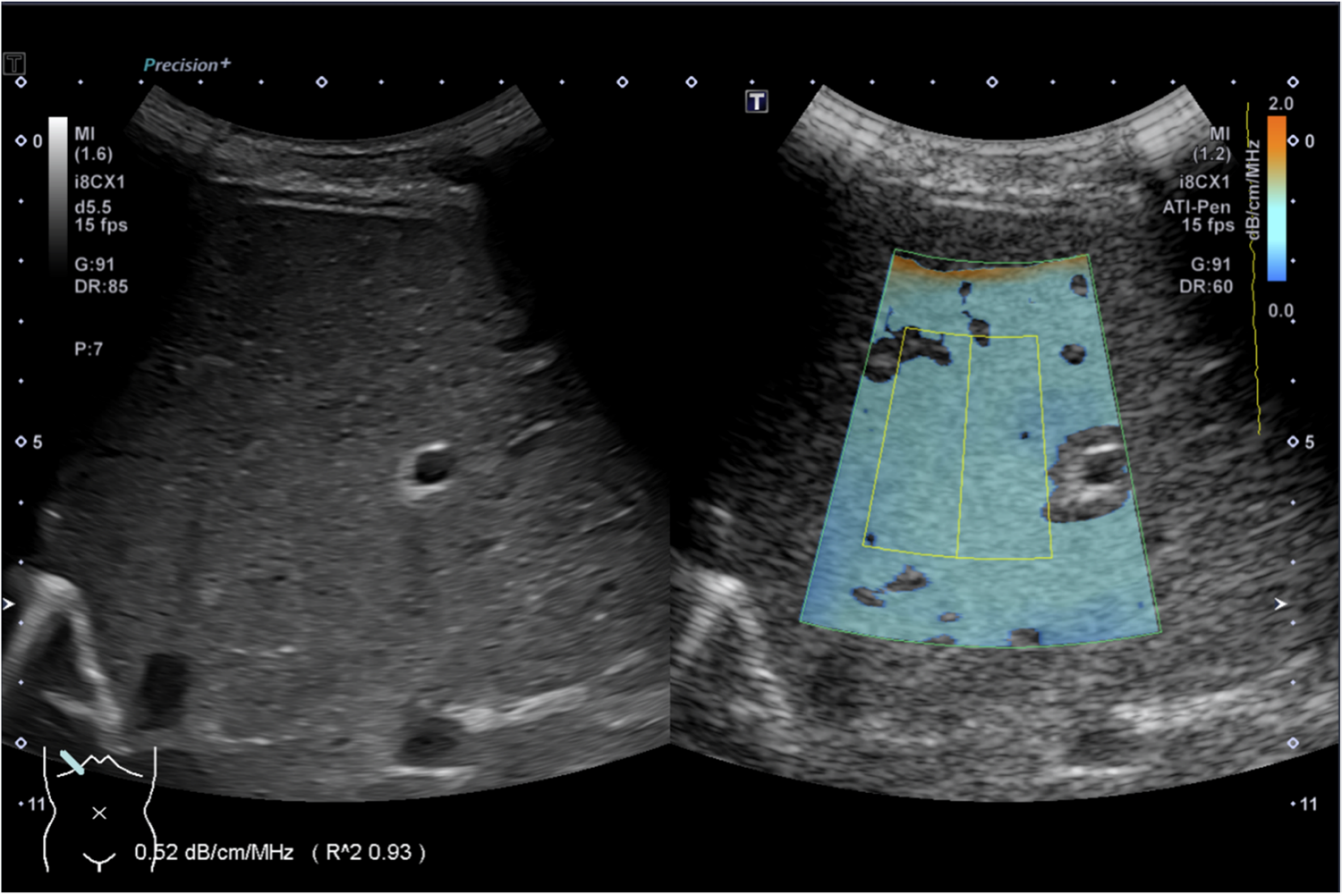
Representative attenuation imaging coefficient measurement (liver ultrasound in the transverse intercostal plane) in a 14-year-old healthy boy with an attenuation imaging coefficient of 0.52 dB/cm/MHz and an *R*^2^ of 0.93
